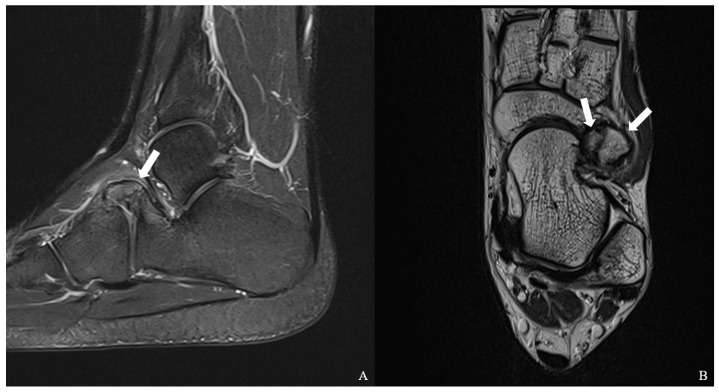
Magnetic resonance imaging of the left foot. (A) Fat-suppressed sagittal T2-weighted image demonstrates focal high signal intensity consistent with bone marrow edema at the calcaneus–ossicle interface (arrow), without a definite fracture line. (B) Coronal T1-weighted image demonstrates two well-defined accessory ossicles adjacent to the anterior process of the calcaneus (arrows), showing smooth cortical margins without articular continuity.
